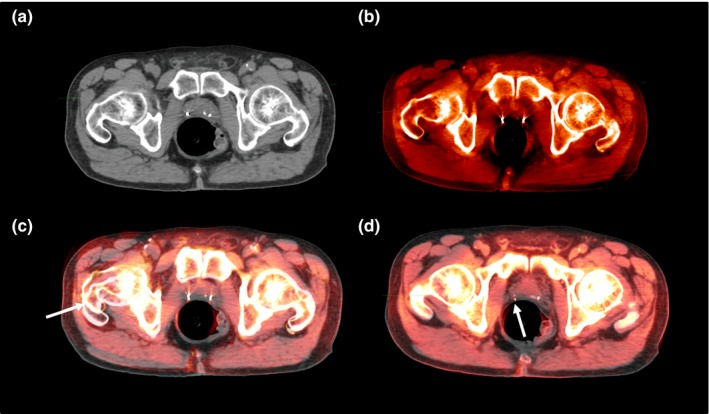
For a selected patient, (a) an axial planning image with two implanted markers; (b) the corresponding axial kV‐CBCT image as (a); (c) marker‐based alignment of images (a) and (b); (d) soft‐tissue based alignment of image (a) and (b). (Arrows indicate the misalignments).
